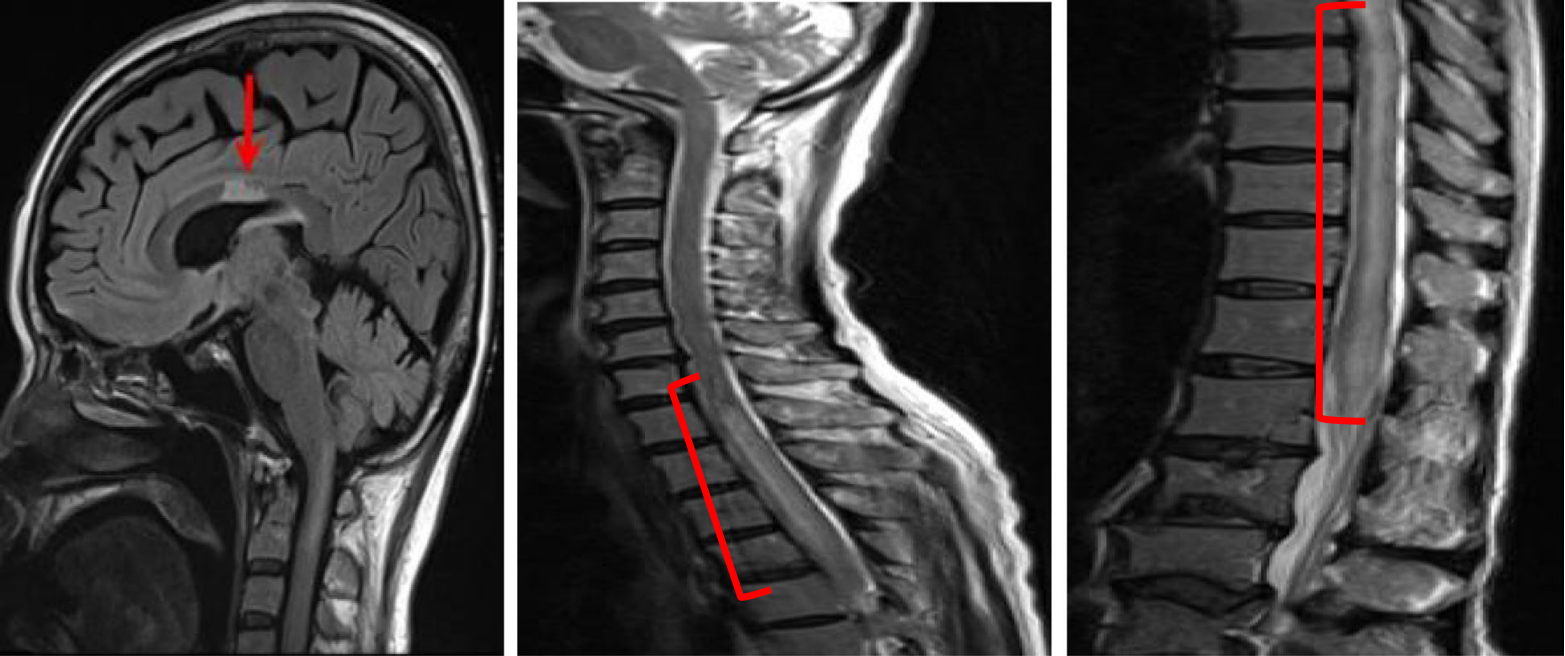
MRI images of the patient’s head and spine. Flair (left panel) and T2-weighed (middle and right panels) images of the patient at the onset of transverse myelitis are shown
